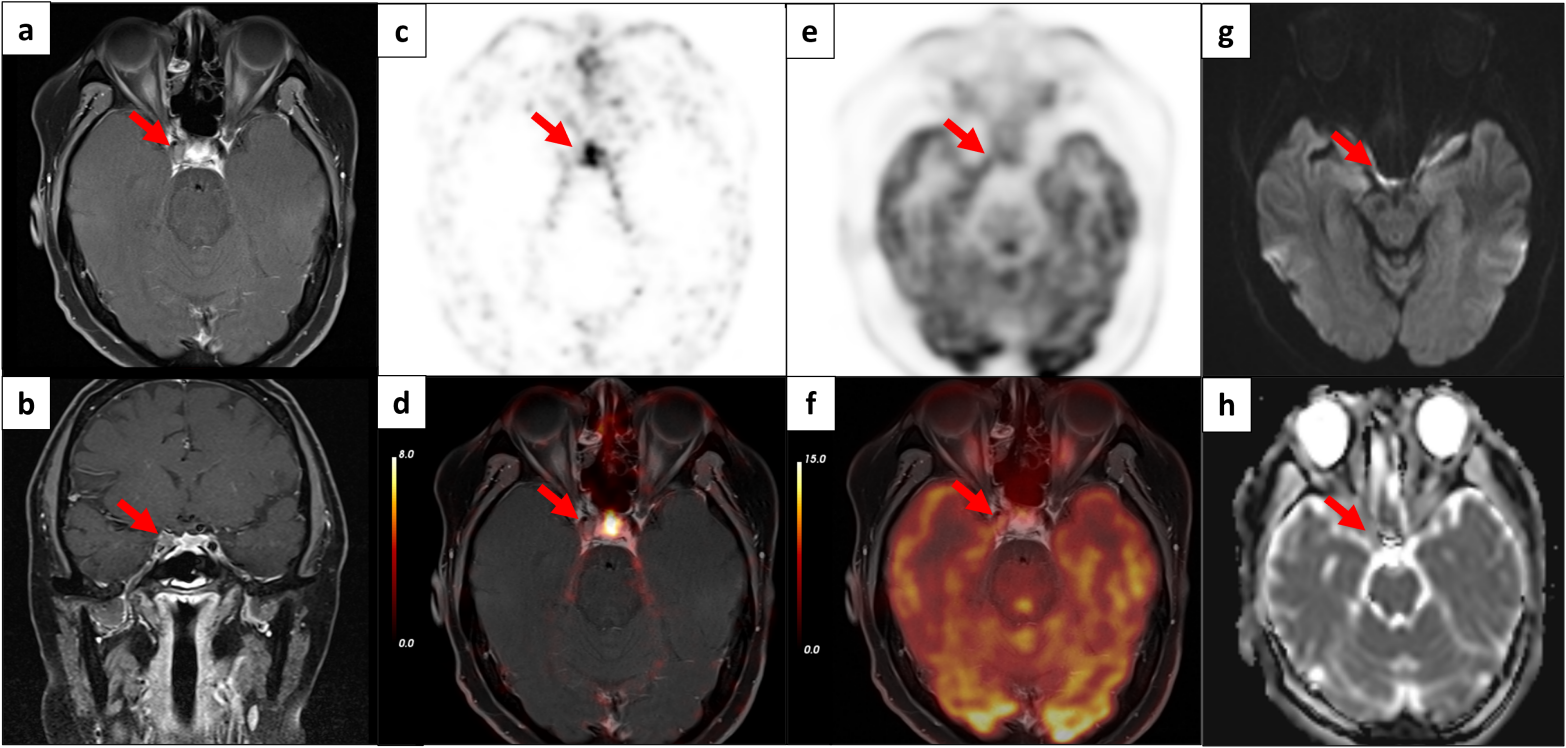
Multimodal PET/MR characterization of a corticotroph adenoma. A 24-year-old woman with CD due to an ACTH-secreting pituitary microadenoma (red arrows). (a) Non-contrast axial T1-weighted MR demonstrates a hypointense lesion in the left sella turcica. (b) Contrast-enhanced coronal T1-weighted MR reveals non-enhancement of the lesion. (c, d) [⁶⁸Ga]Ga-DOTATATE PET and PET/MR fusion images show reduced somatostatin receptor expression in the adenoma (SUVmax 3.60) versus normal pituitary tissue (SUVmax 10.97). (e, f) [^18^F]F-FDG PET and PET/MR fusion images demonstrate increased glucose metabolism in the lesion (SUVmax 10.41) compared to contralateral parenchyma (SUVmax 7.79). (g) Diffusion-weighted imaging (DWI) displays restricted diffusion with hyperintensity. (h) Corresponding ADC map confirms reduced diffusivity (ADCmin 5.1×10⁻⁴ mm²/s vs 11.0×10⁻⁴ mm²/s in normal tissue).
